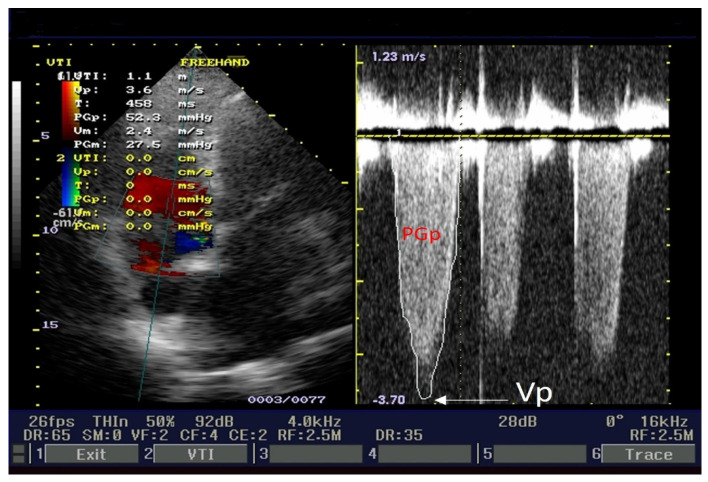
Echocardiography apical 4-chamber view: pulmonary artery systolic pressure determination.
